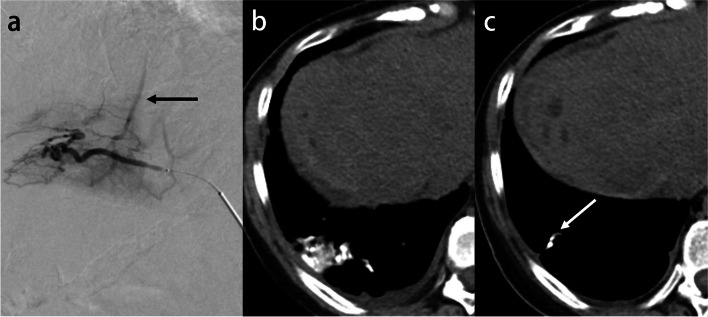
Pulmonary lipiodol embolism following transcatheter arterial chemoembolization for hepatocellular carcinoma in a 54-year-old woman. a A shunt between the right inferior phrenic artery and the hepatic vein (arrow) was revealed during chemoembolization with lipiodol. b Postoperative CT image shows a high-attenuation lesion in the right lower lobe, which is compatible with the accumulation of lipiodol. c Three months later, the dense lesion was smaller and had changed into a linear-like opacity (arrow)
